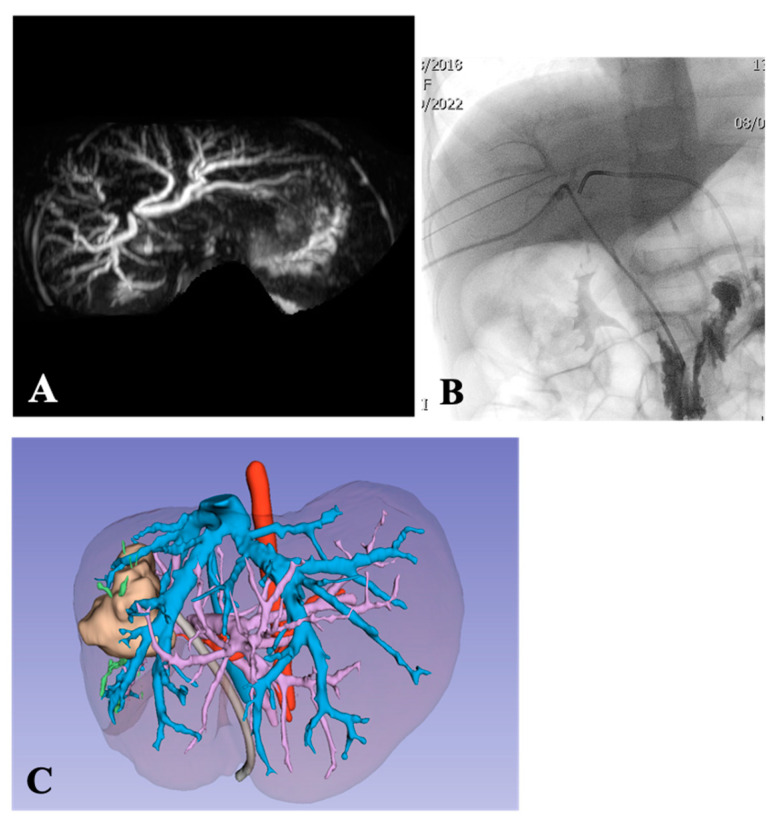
(A): Cholangio MRI of patient n. 12 showed a sizeable hepatic lesion of the right lobe extended from the subcapsular region to the hilum and determined biliary tree compression. The vascular branches form an intricate “caput medusae.” (B): Combined approach with percutaneous cholangiography and ERCP. The combined approach (Randez-vous) permitted to insert of a guidewire (0.035–400 mm), allowing a balloon dilatation (6 mm) and the stenting (8.5 F–12 cm). (C): VR reconstruction after removal of the left biliary stent. The intrahepatic right biliary tree could not be fully visualized due to complete obstruction of the anterior and posterior segments.
